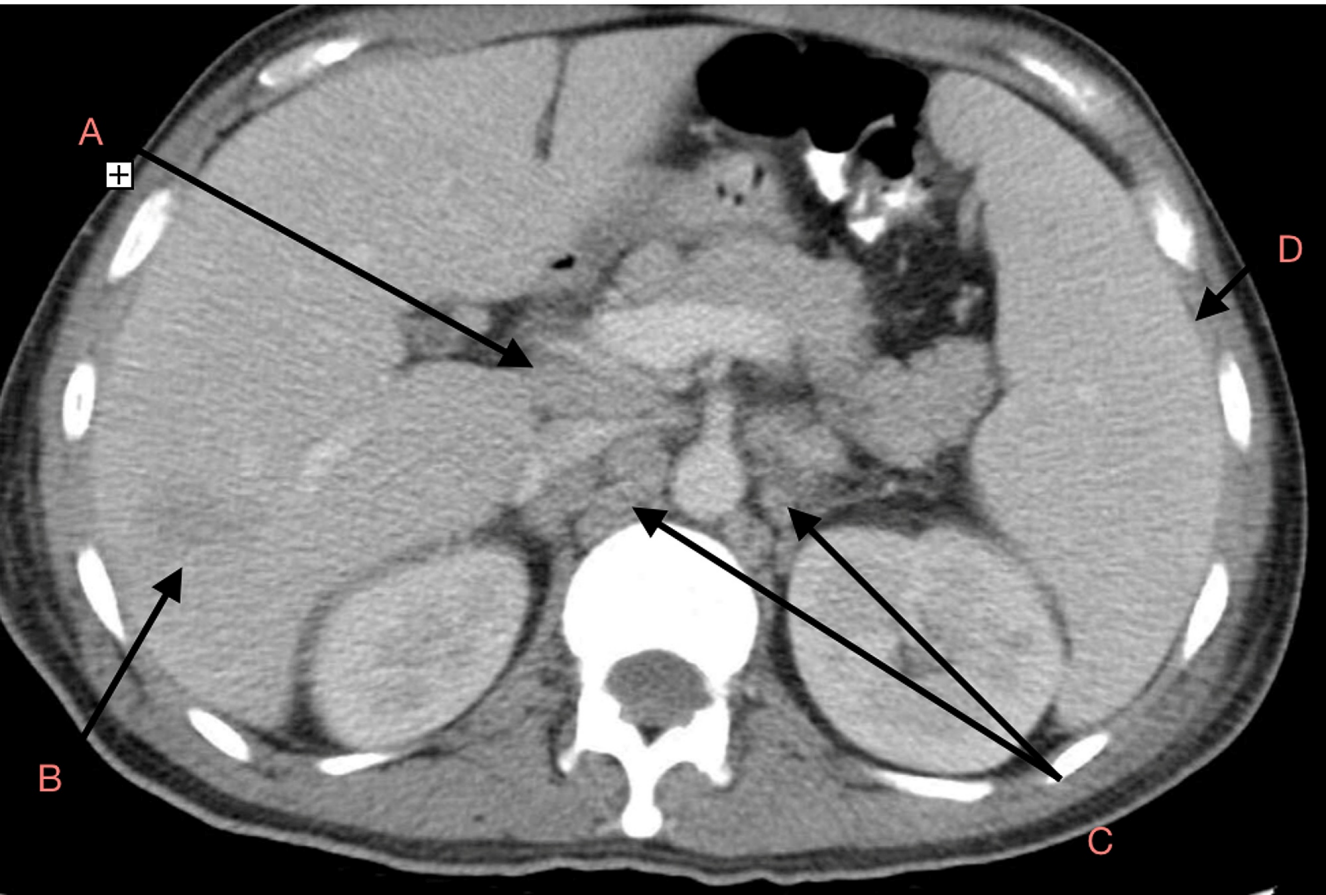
CT abdomen showing hypodense lesion in the liver, splenomegaly and retroperitoneal lymphadenopathy A: Portocaval Lymph Nodes, B: Right Posterior Hepatic Lobe Hypodense Lesion, C: Paraaortic Retroperitoneal Lymphadenopathy, D: Enlarged Spleen
